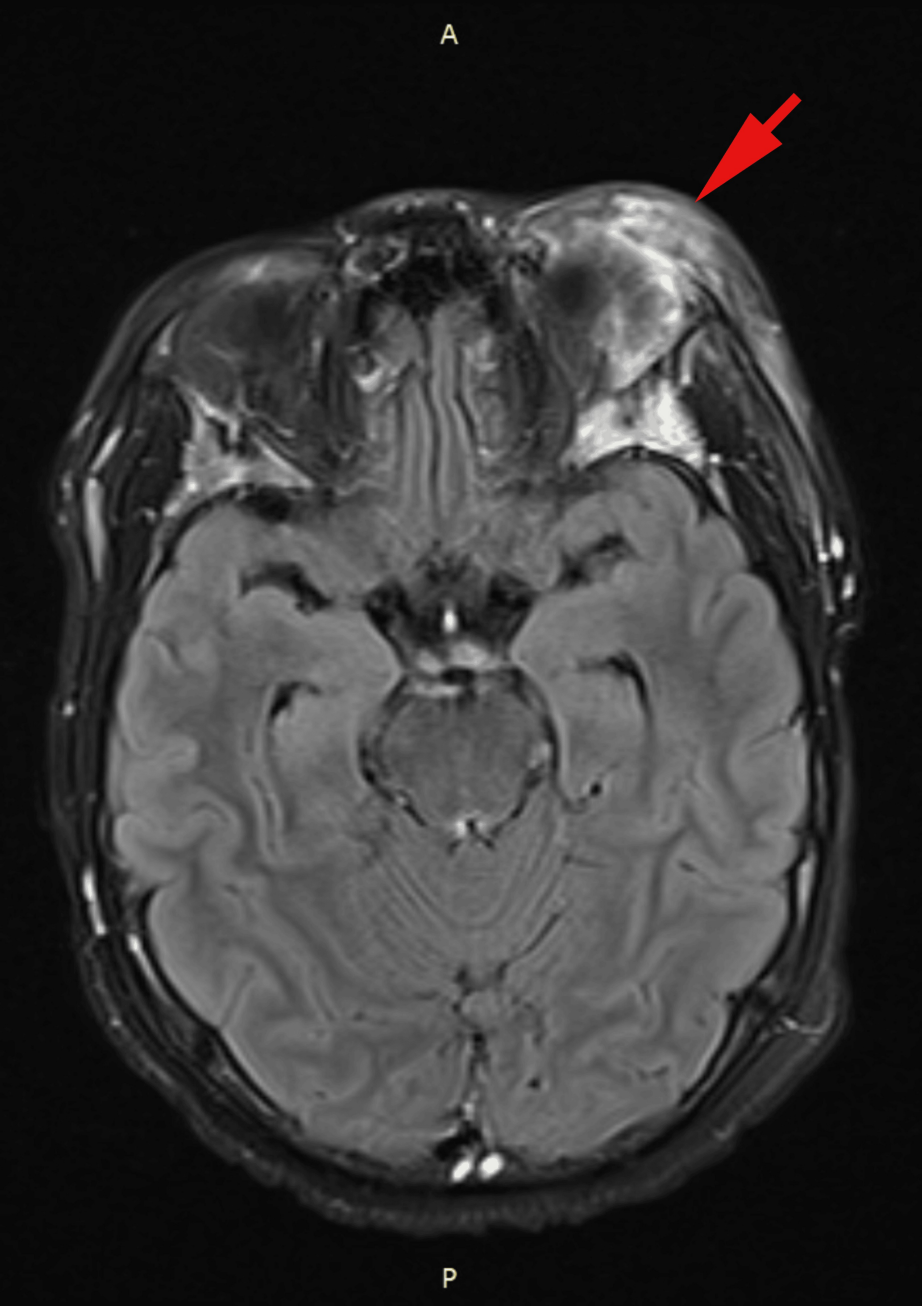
Post-contrast Axial T2 fluid-attenuated inversion recovery (FLAIR) MRI Axial T2 FLAIR post-contrast MRI demonstrating extensive left-sided preseptal and postseptal orbital abscesses with associated dacryoadenitis of the left lacrimal gland and diffuse infectious phlegmon extending across periorbital tissues. Bone marrow edema in the frontal calvarium and greater sphenoid wings is evident, suggesting osteomyelitis. Right maxillary sinus opacification indicative of sinusitis is also present.
